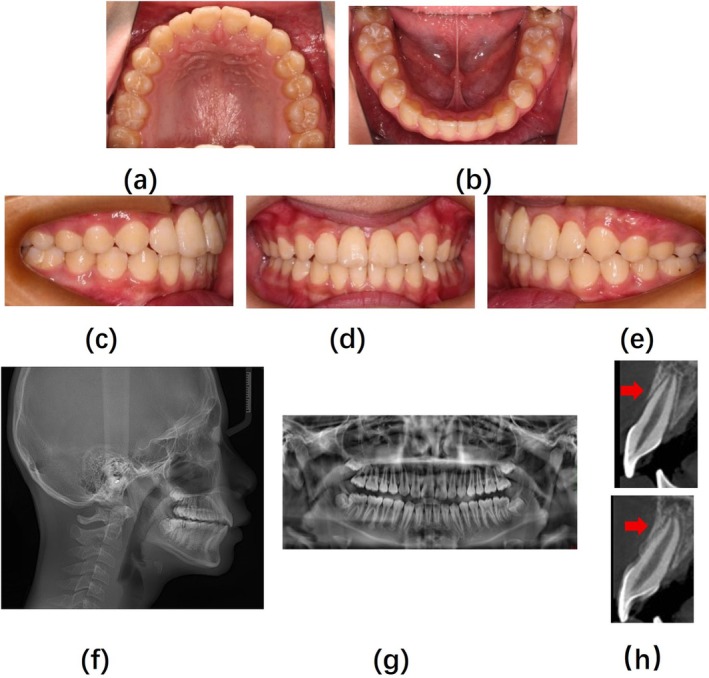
Clinical and radiograph view after orthodontic treatment. (a–e) Intraoral views after orthodontic treatment; (f–h) radiograph views after orthodontic treatment.
